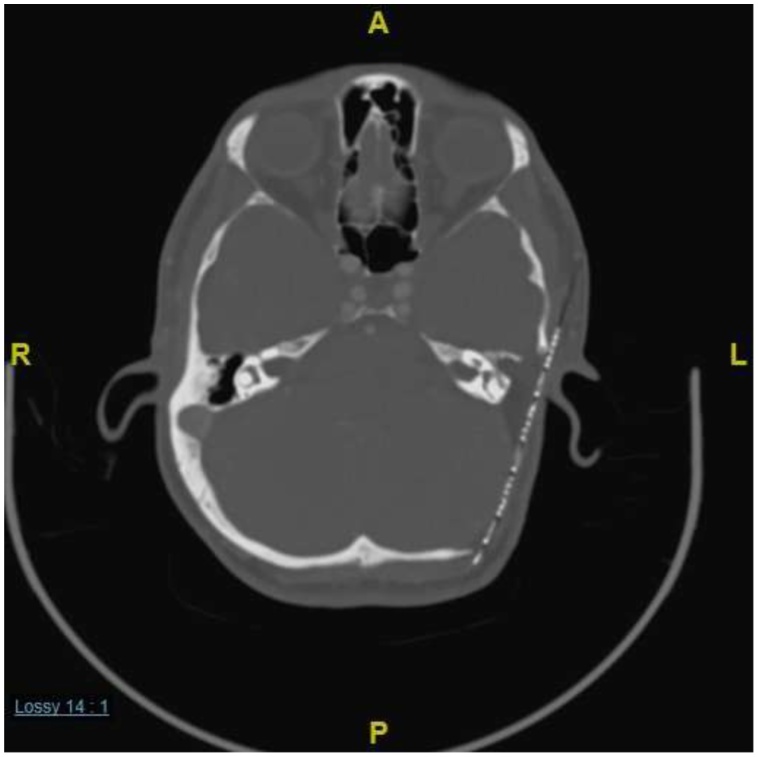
CT axial cut post resection of left temporal cholesterol granuloma.
